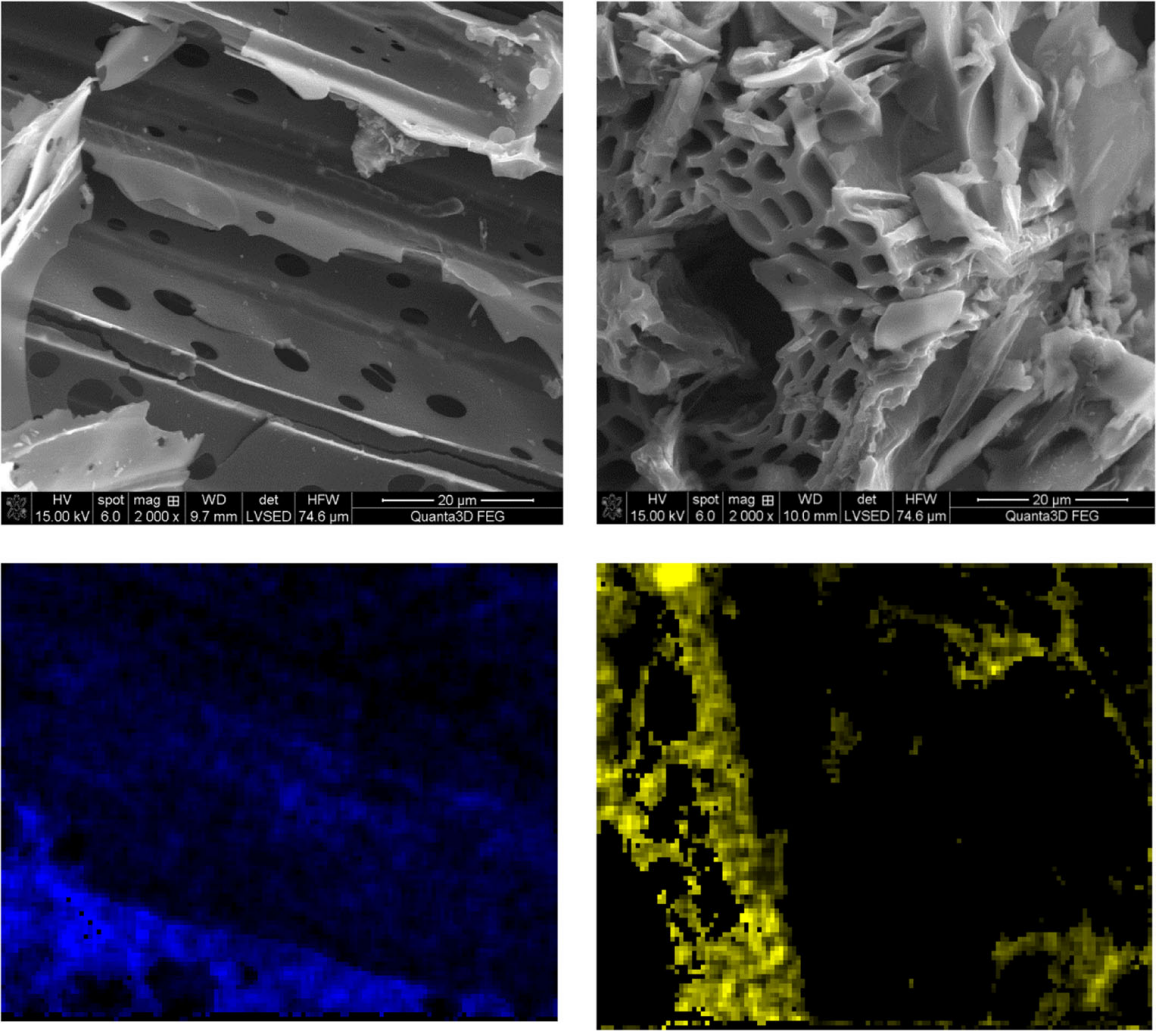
SEM of *1* BCS and *2* BCW materials used in Cr(VI) adsorption processes and X-ray elemental mapping. *Blue* and *yellow* colors show Cr loaded on particular BCs
